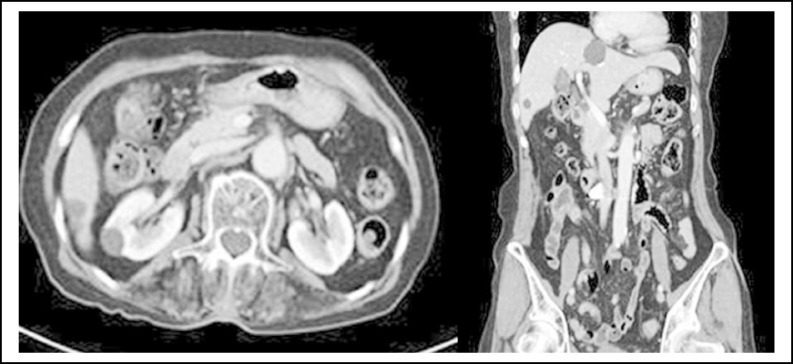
Eight weeks after anticoagulation therapy, computed tomography revealing decompression of the inferior vena cava and disappearance of inferior vena cava thrombosis
